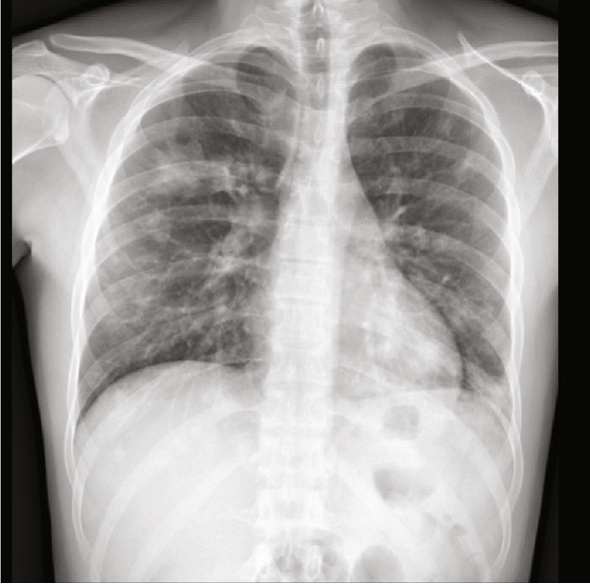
Chest x-ray with bilateral pulmonary consolidations.
